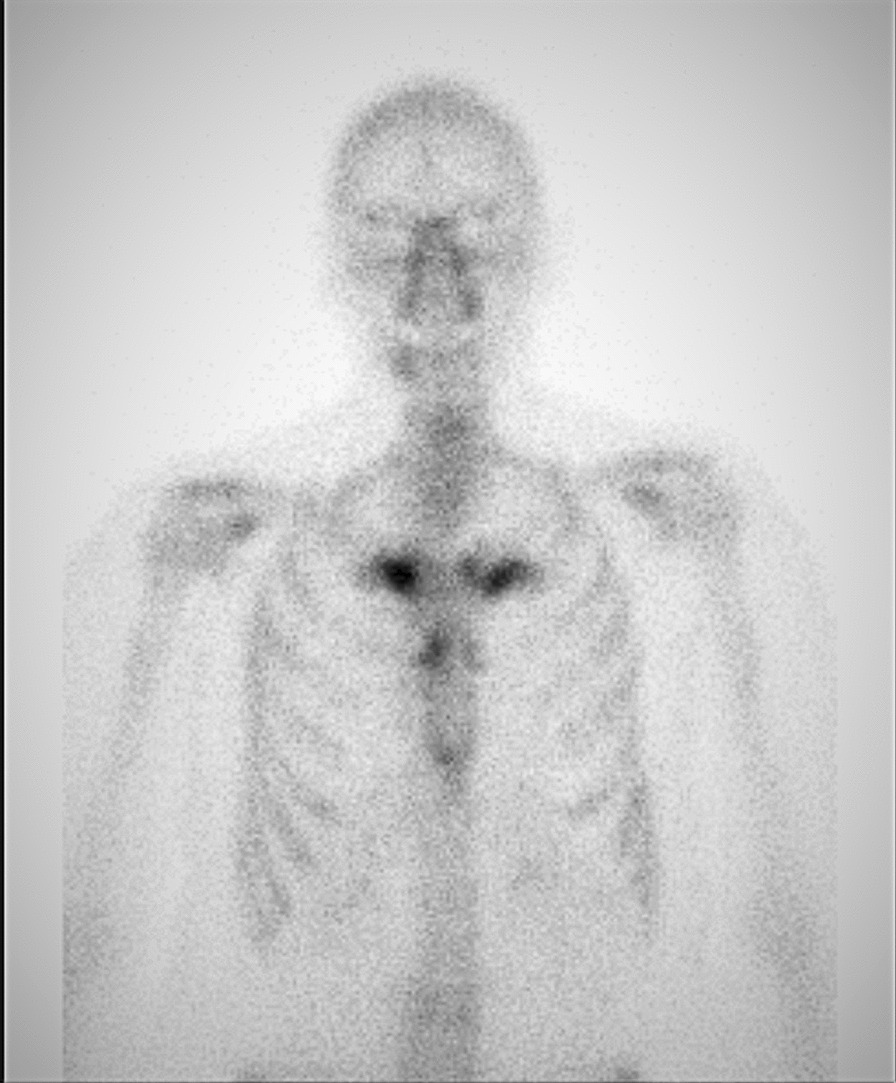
Bone scintigraphy shows increased uptake in the manubrium and sternoclavicular joints
